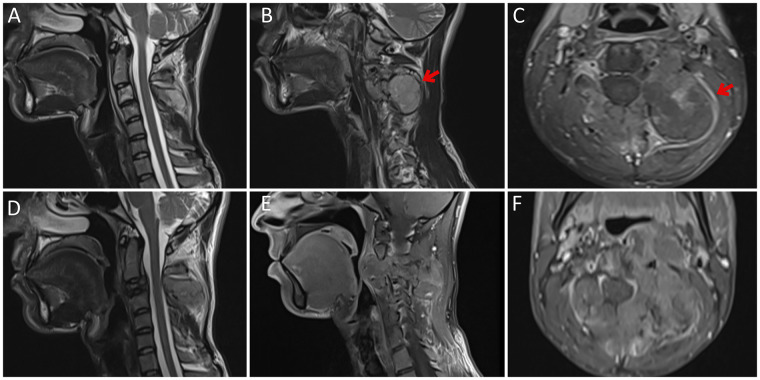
Cervical magnetic resonance imaging (MRI) in March and April 2018. In March 2018, (A) a sagittal T2-weighted image demonstrates preserved cervical cord caliber and signal without an intraspinal mass; (B) an off-midline sagittal T2-weighted image shows a left posterior paraspinal upper cervical mass (red arrow); and (C) an axial post-contrast T1-weighted image demonstrates peripheral enhancement of the lesion extending toward the left C3 neural foramen (red arrow). In April 2018, (D) a midline sagittal T2-weighted image demonstrates interval progression with vertebral body collapse at the C3 level; (E) an off-midline sagittal post-contrast T1-weighted image shows expansion of the paraspinal mass with anterior extension toward the retropharyngeal region; and (F) an axial post-contrast T1-weighted image demonstrates mass effect with narrowing of the pharyngeal airway.
